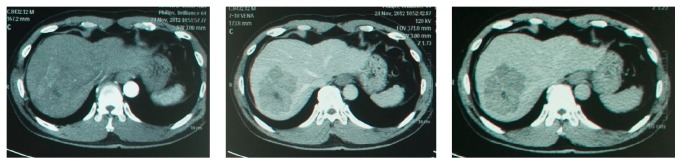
Arterial, venous, and delayed phase of Abdominal CT Scan after diagnosis (November 2012).
